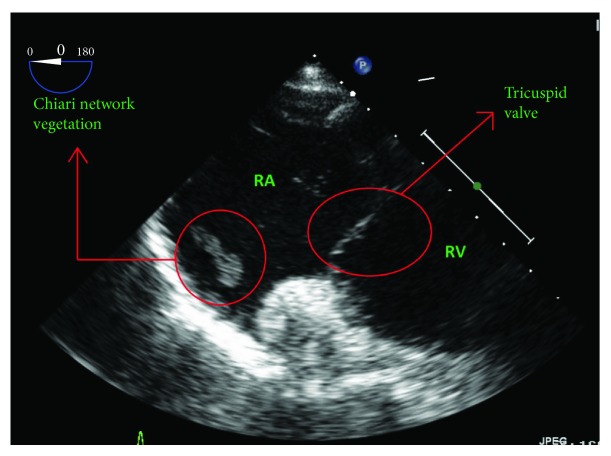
Transesophageal echocardiogram image showing the Chiari network vegetation separated from the tricuspid valve.
